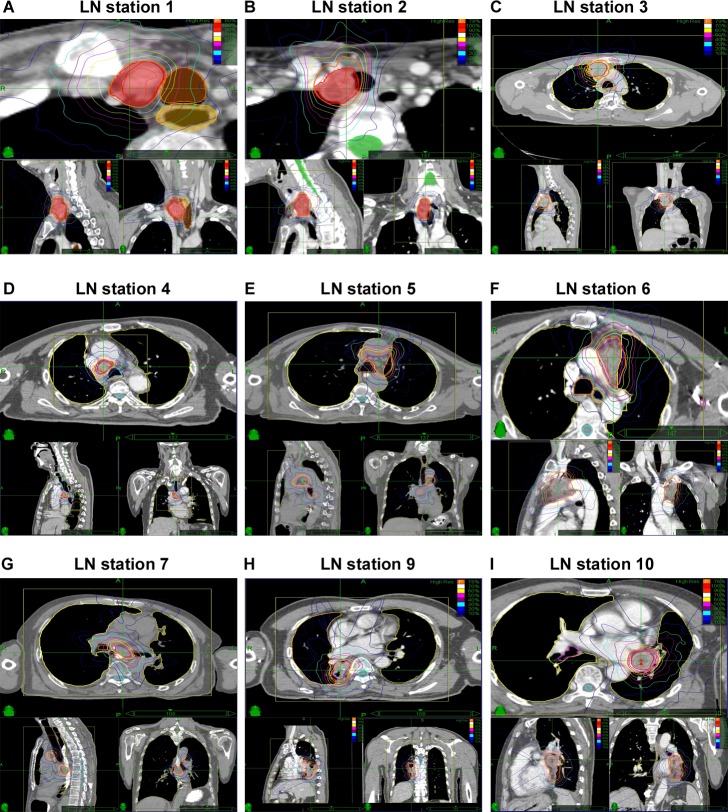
Representative planning CT and isodose distributions with SRT of patients with R/SP-MLNMs originating from NSCLC The purple and yellow lines indicate GTV and PTV, respectively. (A-I) The LN stations of MLNMs. CT: computer tomography; SRT: stereotactic radiotherapy; R/SP-MLNM: recurrent / second primary mediastinal lymph node metastases; GTV: gross tumor volume; PTV: planning target volume.
